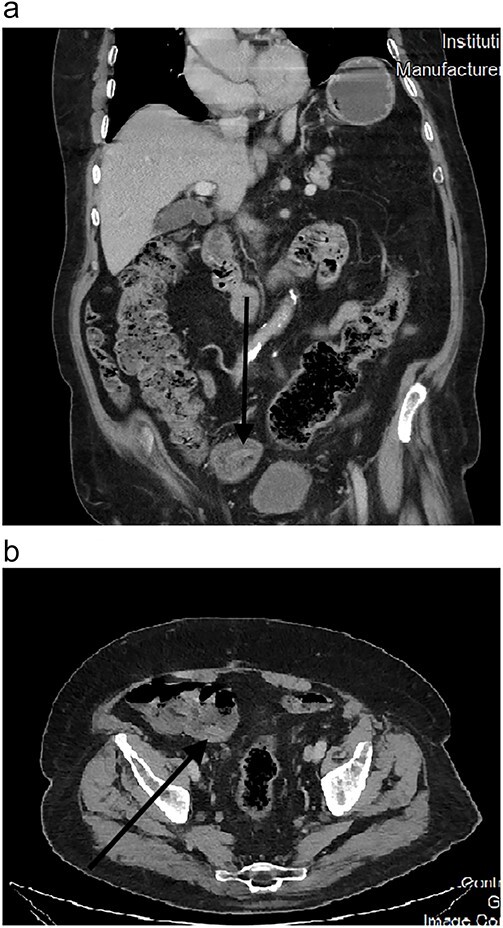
(a) CT scan February 2023. (b) CT scan February 2023.
